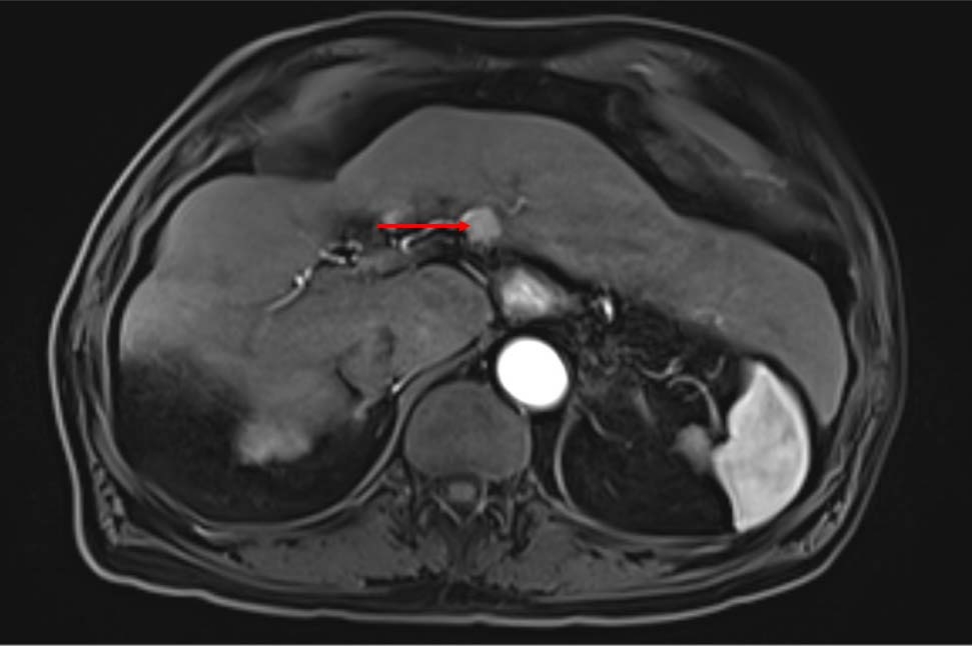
Abdominal magnetic resonance imaging with a 1.6 × 1.4 cm arterially enhancing lesion in the segment 3.
